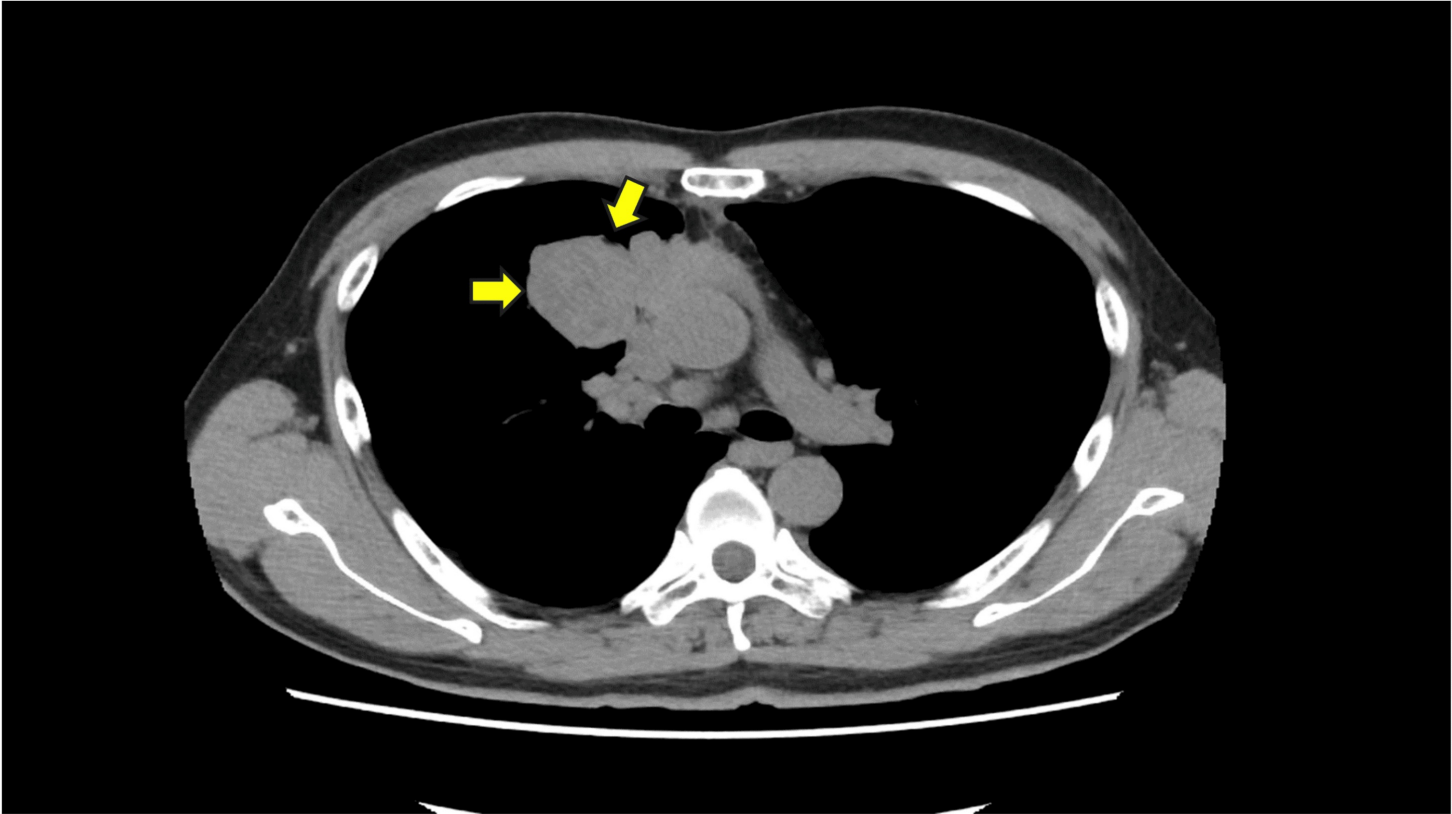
Thymoma on chest CT. Thymoma is found in the anterior mediastinum extending to the right lung (arrows).
